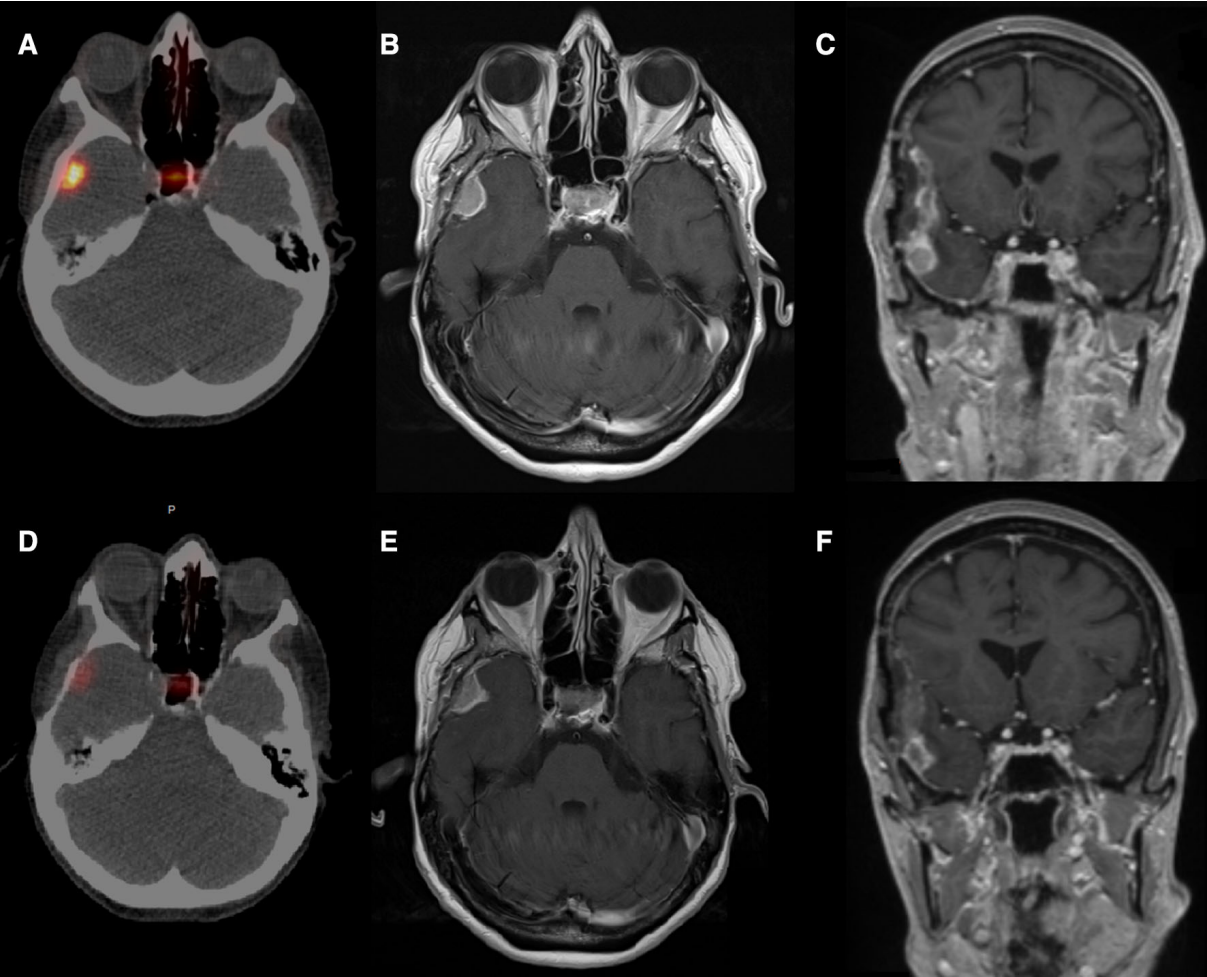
A Pre-treatment gallium-68-DOTATOC PET/CT showing high somatostatin receptor expression in the right temporal meningioma and physiological expression in the pituitary gland. B + C Pre-treatment gadolinium enhanced T1 MRI sequence showing a homogeneously enhancing solid lesion. D Post-treatment gallium-68-DOTATOC PET/CT showed 79% decrease in SSTR2 expression. E + F Gadolinium enhanced T1 MRI sequence showed a partial response of the right temporal meningioma, − 38% in volume and − 24% in longest diameter, and additionally central necrosis of the tumour can be acknowledged on the coronal reconstruction (F) and reduction in enhancement of tumour deposits in the old resection cavity (F)
